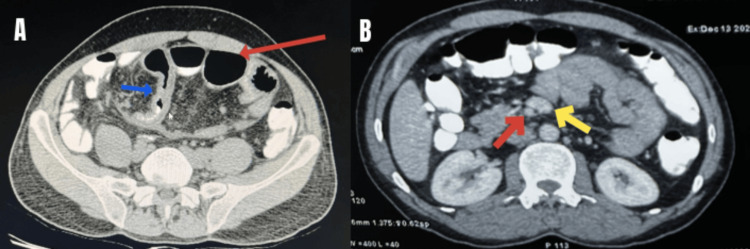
CT abdomen in the axial plane (A) showing dilated bowel loops (red arrow) and thickened bowel loops (blue arrow) in the cocoon (B) superior mesenteric artery (red arrow) seen to the right of the superior mesenteric vein (yellow arrow) suggestive of partial malrotation
